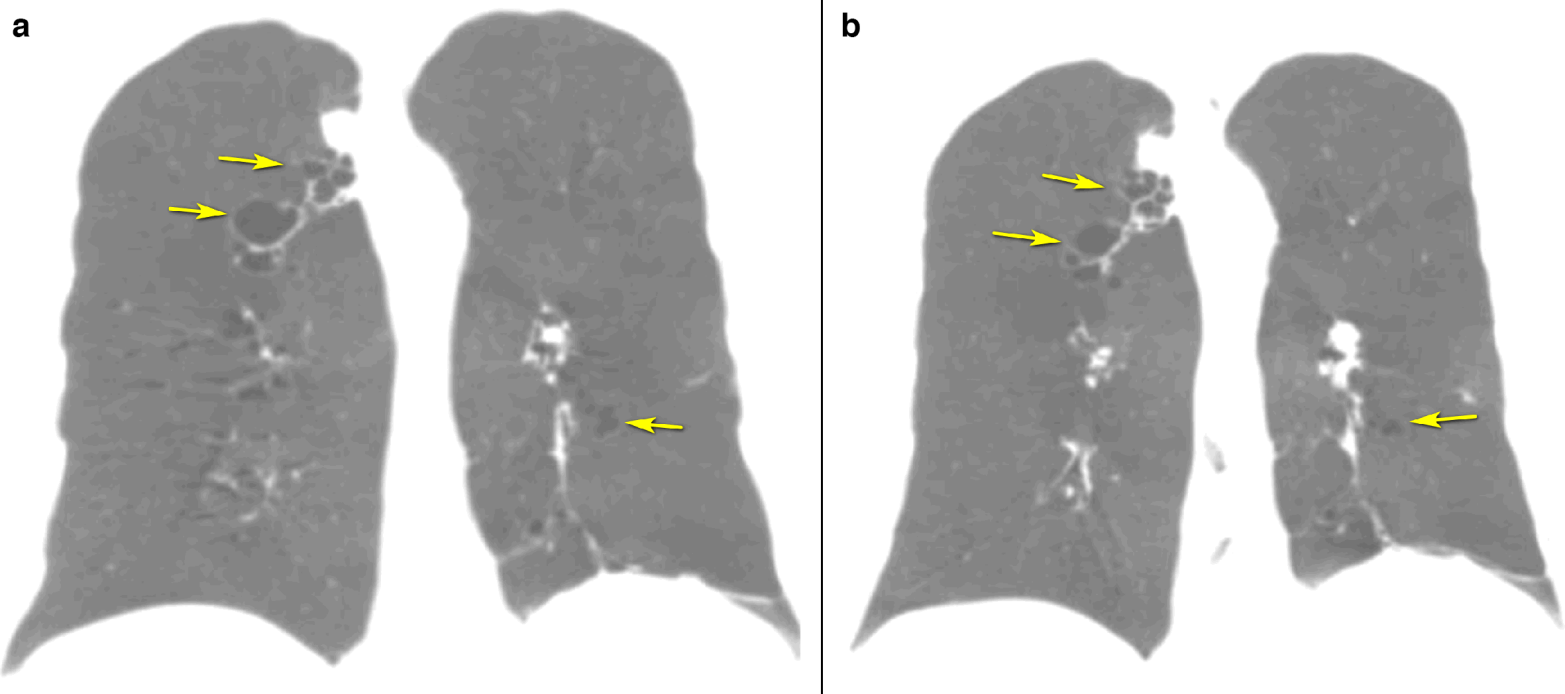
A. Coronal CT scan of the chest with minimal intensity projection (MinIP) in inspiration showing cystic bronchiectasis in fourth- to sixth-order bronchi in the right upper and left lower lobes with calcified hilar lymph nodes on the left- and right-sided aortic arch. B. Coronal CT scan of the chest with MinIP in the expiratory phase, demonstrating the collapse of the bronchi, a typical finding in this disease.
